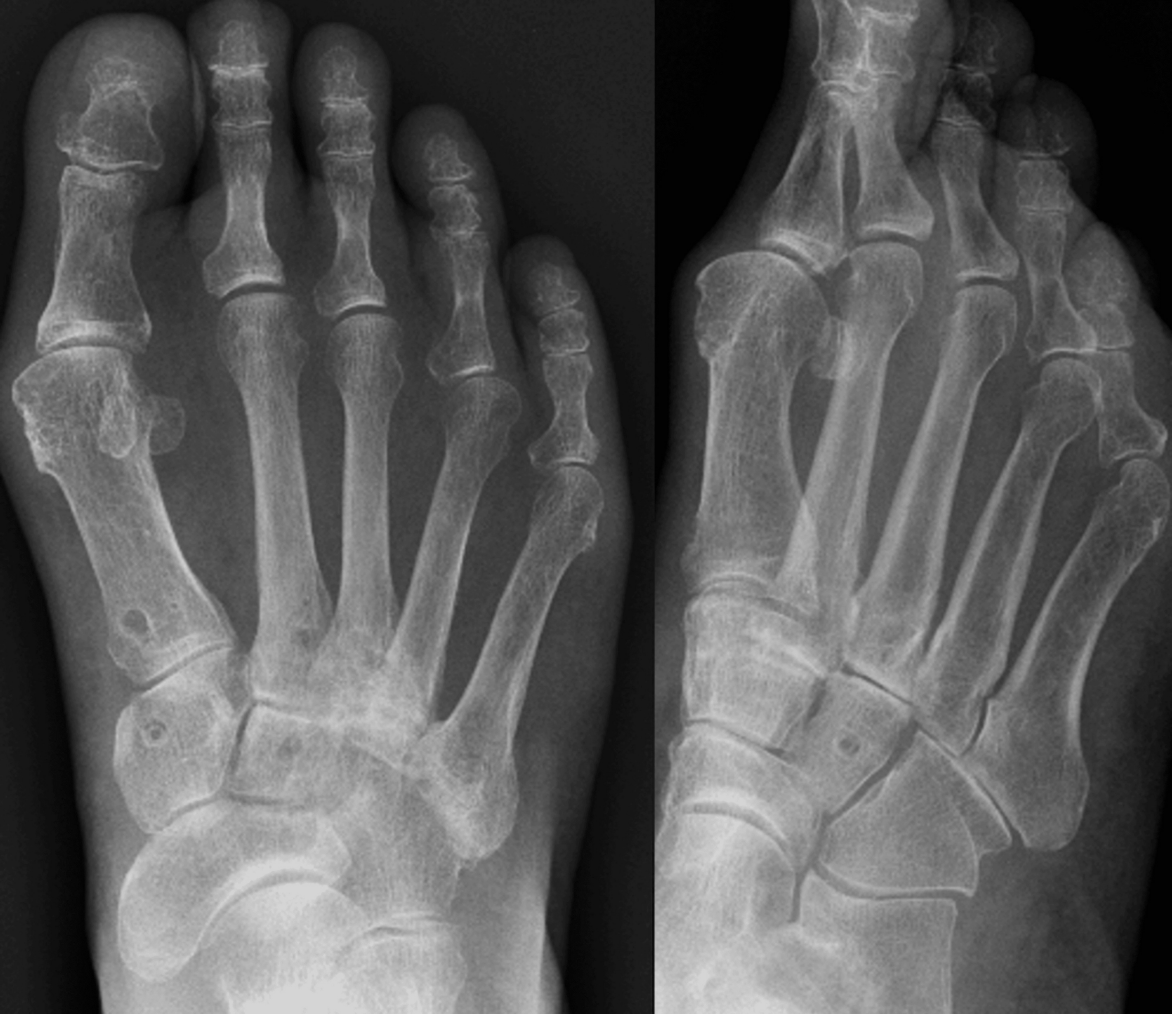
Postoperative X-ray Absorbable screws were carefully inserted into each bone to maintain a moderate tension on the tapes. The fixation of the screw was very secure and stable.
